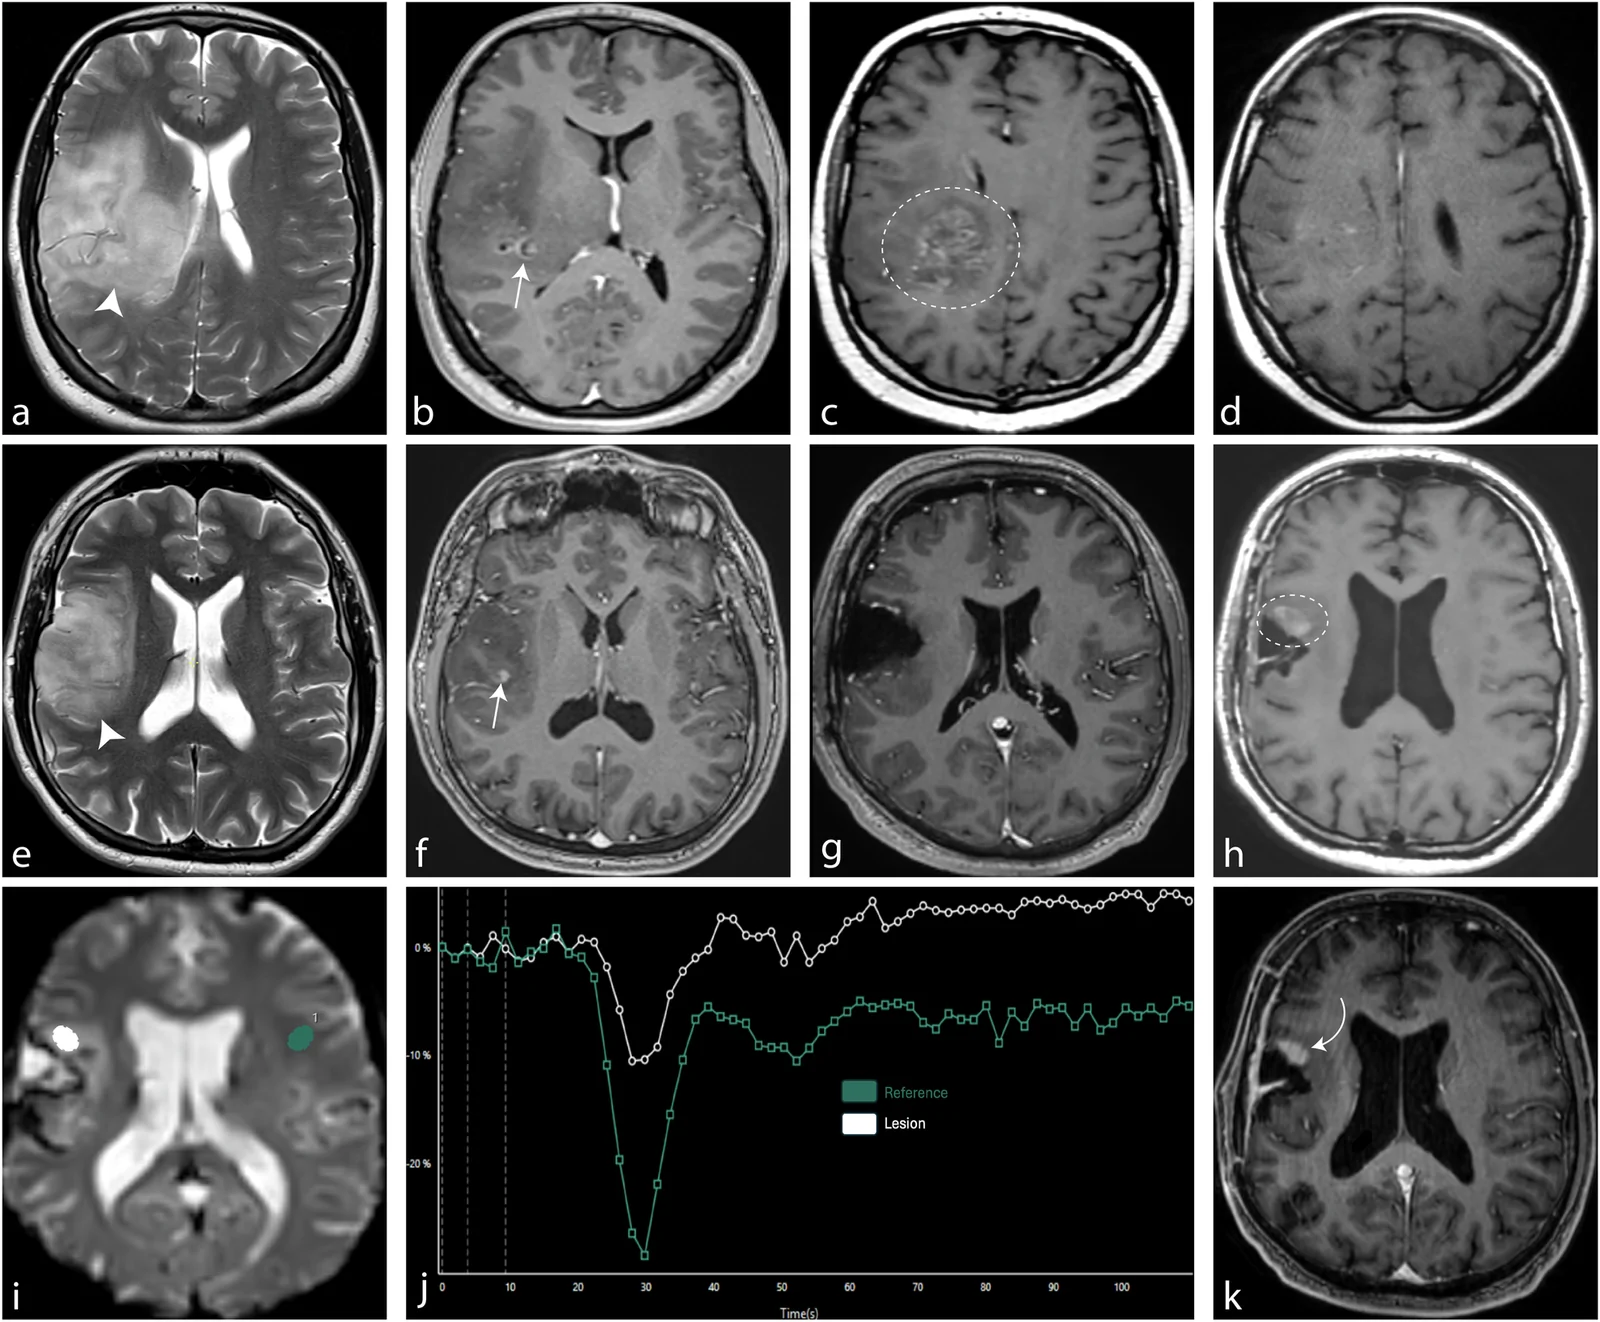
Pseudoprogression after chemoradiotherapy in IDH-mutant astrocytoma, CNS WHO grade 4. Two patients with IDH-mutant astrocytoma, CNS WHO grade 4, treated with radiotherapy and concurrent and adjuvant temozolomide. Case 1 (a–d). a Preoperative axial T2-weighted image showing a right hemispheric mass (arrowhead). b Preoperative axial post-contrast T1-weighted image showing minimal ring enhancement (arrow). c Axial post-contrast T1-weighted image 3–4 months after completion of radiotherapy, showing a clumpy, heterogeneous, Swiss-cheese-like enhancing focus at the tumour site (dashed circle). d Axial post-contrast T1-weighted follow-up shows almost total resolution of enhancement. Case 2 (e–k). e Preoperative axial T2-weighted image showing a right frontal mass (arrowhead). f Preoperative axial post-contrast T1-weighted image showing minimal enhancement (arrow). g Immediate postoperative axial post-contrast T1-weighted image showing the resection cavity without residual nodular enhancement. h Axial post-contrast T1-weighted image 2 months after completion of radiotherapy, showing new nodular enhancement at the anterior margin of the resection cavity (dashed oval). i Dynamic susceptibility contrast (DSC) perfusion source image showing the regions of interest used for analysis: the enhancing lesion (white) and contralateral normal-appearing white matter (teal, labelled 1). j Corresponding time–signal intensity curves, colour-coded as shown in the key within the image: the lesion curve shows a shallower signal drop with prompt return towards baseline compared with the reference curve. k Follow-up axial post-contrast T1-weighted image showing a mild reduction in size of the enhancing focus (curved arrow)
